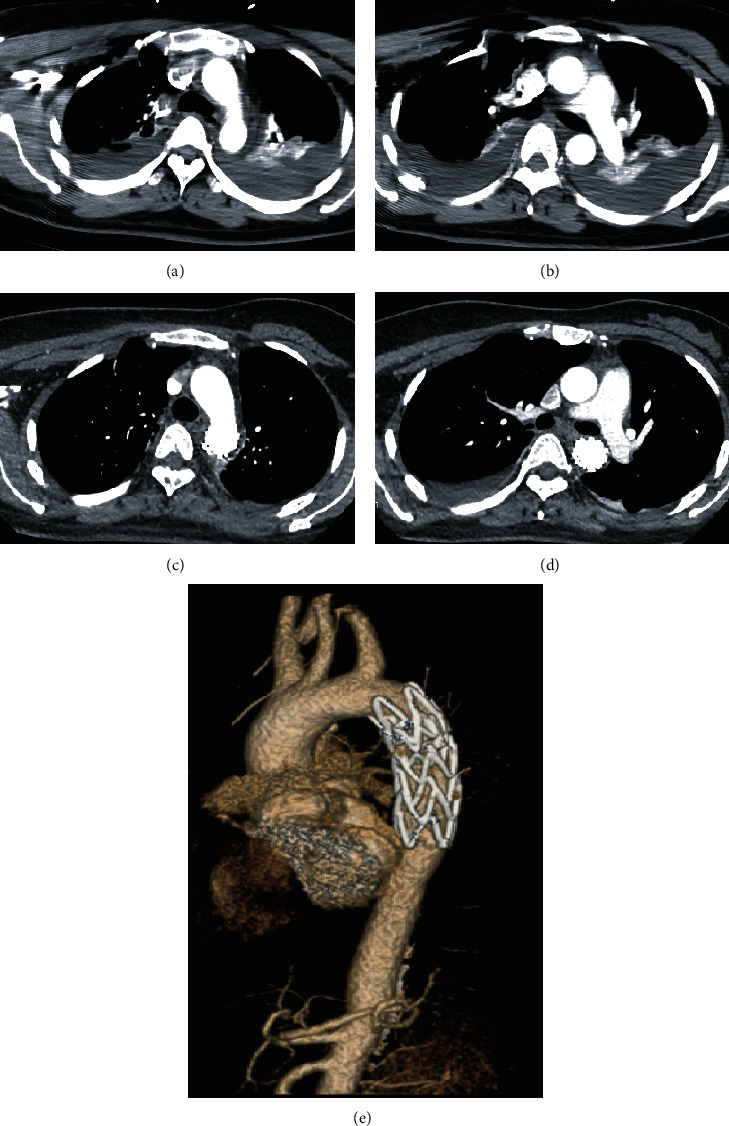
(a and b) CT angiography scan revealed double-lumen aortic arch structure. (b) Percutaneous femoral arteriography revealed a small incision with leaking of the contrast medium of the descending thoracic aorta at the level of the pulmonary artery. (d and e) After aortic stenting, no internal leakage was visible.
